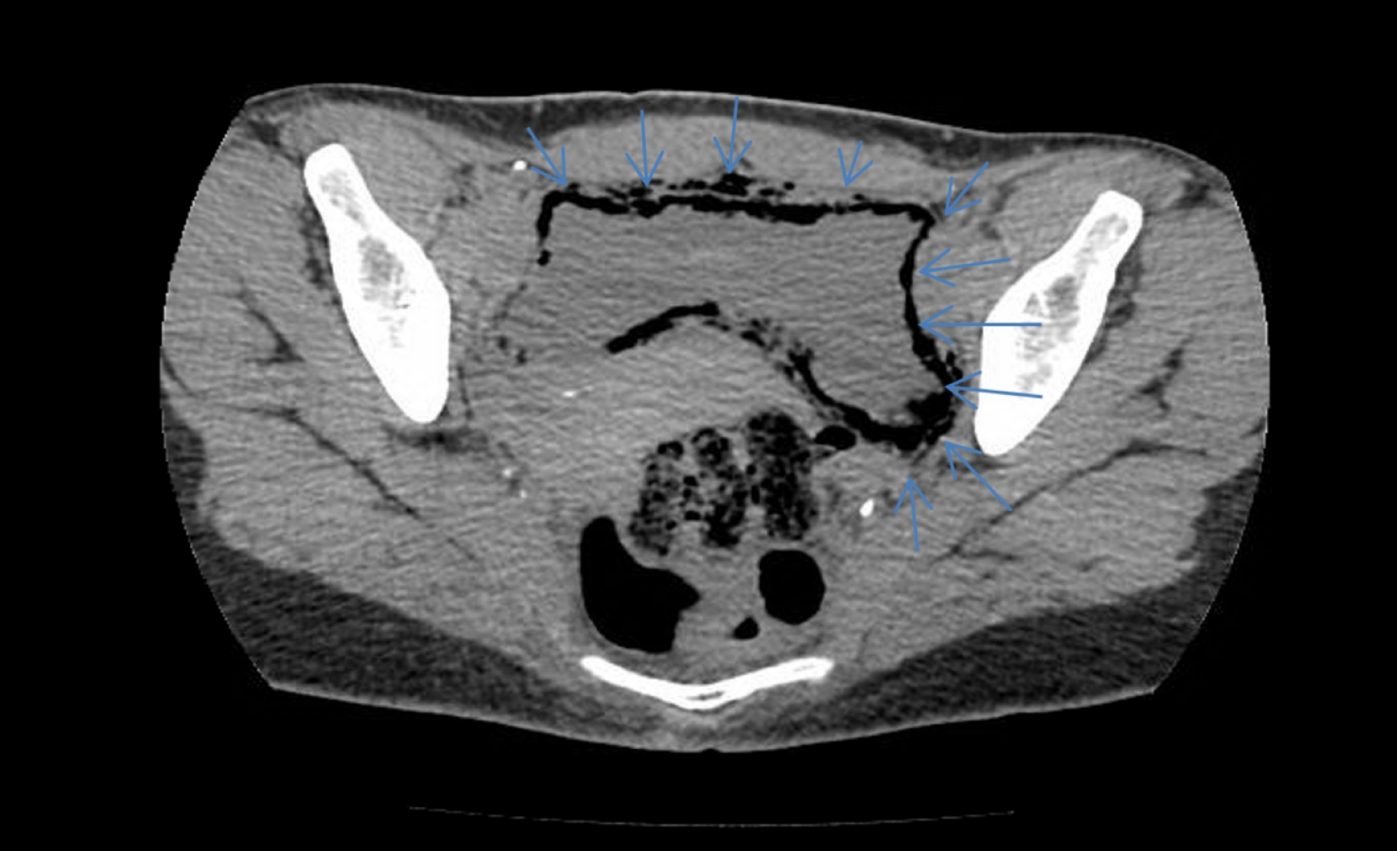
A CT scan of the pelvis and abdomen without contrast indicating abnormal bladder wall thickening that contained air
